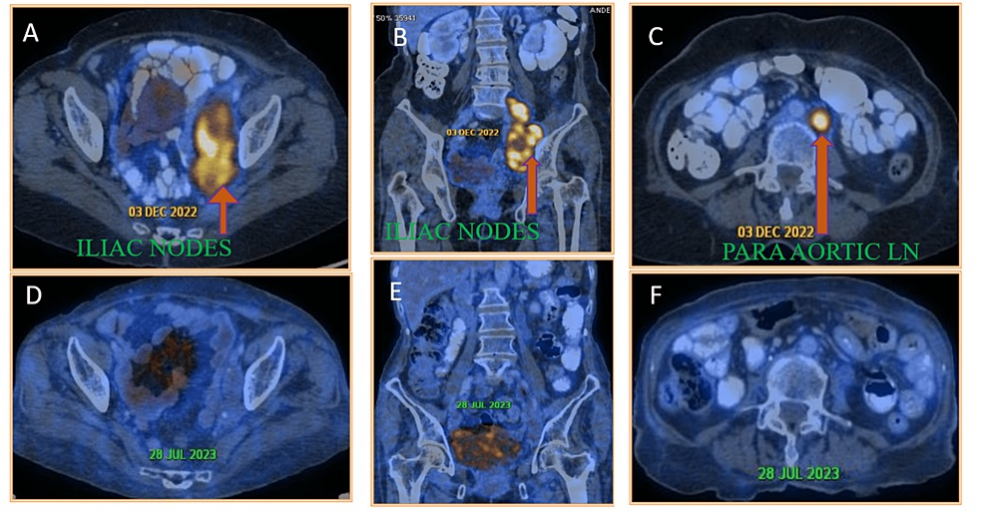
(A, B, C) Post-treatment PET-CT showing significant reduction in iliac nodes (40x15mm) with low SUV uptake (2.3). (D, E, F) Complete resolution of left lower para-aortic nodes.
